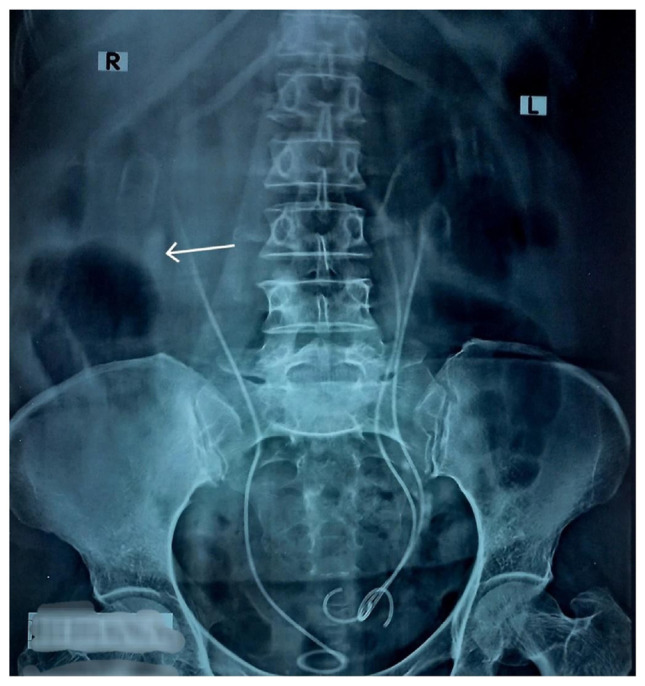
Postoperative plain x-ray kidney-ureter-bladder (KUB) anteroposterior (AP) view. This postoperative plain radiograph is confirming the correct positioning of all three DJ stents, complete clearance of left ureteric and renal calculi and an intact right renal calculus as indicated by a white arrow.
